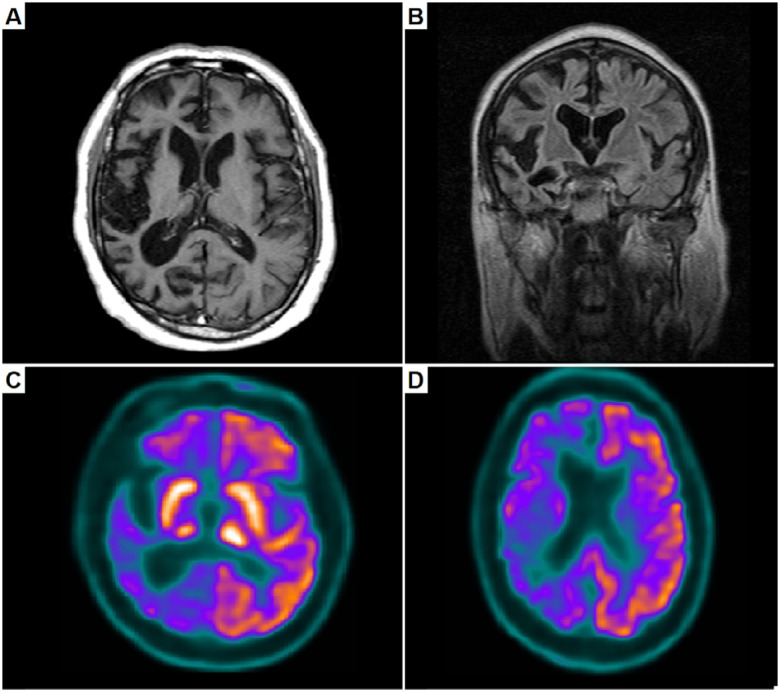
Brain magnetic resonance imaging – T1 axial (A) and coronal (B) reveal right-side atrophy; ^18^F-FDG PET-CT (C and D) shows right-side hypometabolism.
